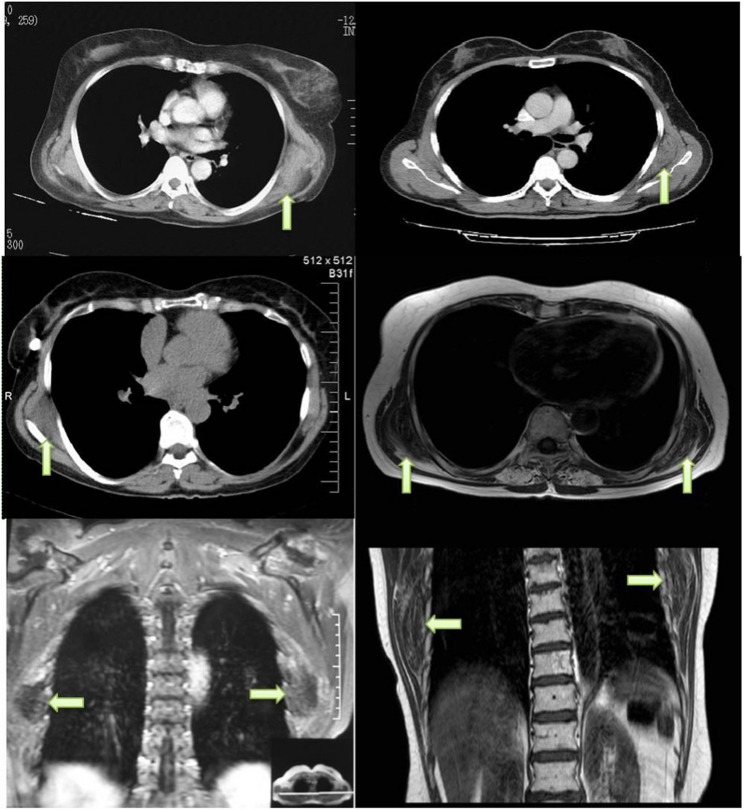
Representative computed tomography (CT) and magnetic resonance imaging (MRI) scans of elastofibroma dorsi. Axial CT images (upper and middle rows, left panels) show poorly encapsulated, soft-tissue masses located between the lower scapula and posterior chest wall (arrows). The lesions demonstrate heterogeneous attenuation with interspersed fat density strands. Corresponding axial, coronal, and sagittal T1- and T2-weighted MR images (right panels) reveal a well-defined, heterogeneous mass with alternating fibrous (low signal) and fatty (high signal) areas, characteristic of elastofibroma dorsi
